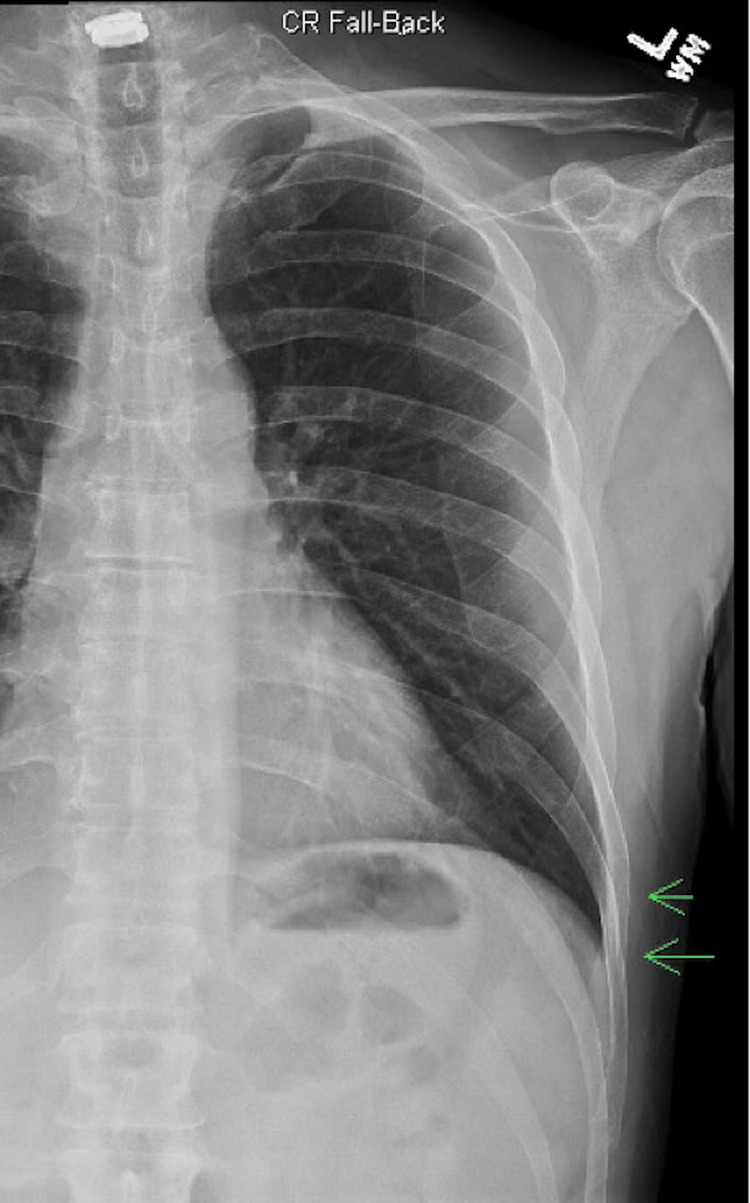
Chest radiograph demonstrating two rib fractures on the left (arrows)
